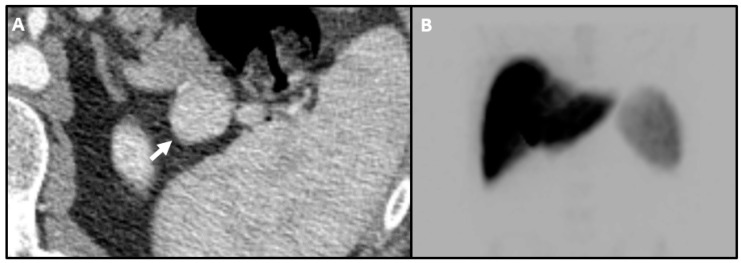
Mass adjacent to pancreatic tail. (A) Axial CT image shows an ambiguous enhancing mass (arrow). It is unclear whether this mass is intra- or extra-pancreatic and splenule was raised as a possibility. (B) Frontal planar ^99m^Tc-Sulfur Colloid image without focal uptake in the region of the pancreatic tail, making splenule unlikely. This mass was surgically excised and confirmed to be an insulinoma.
